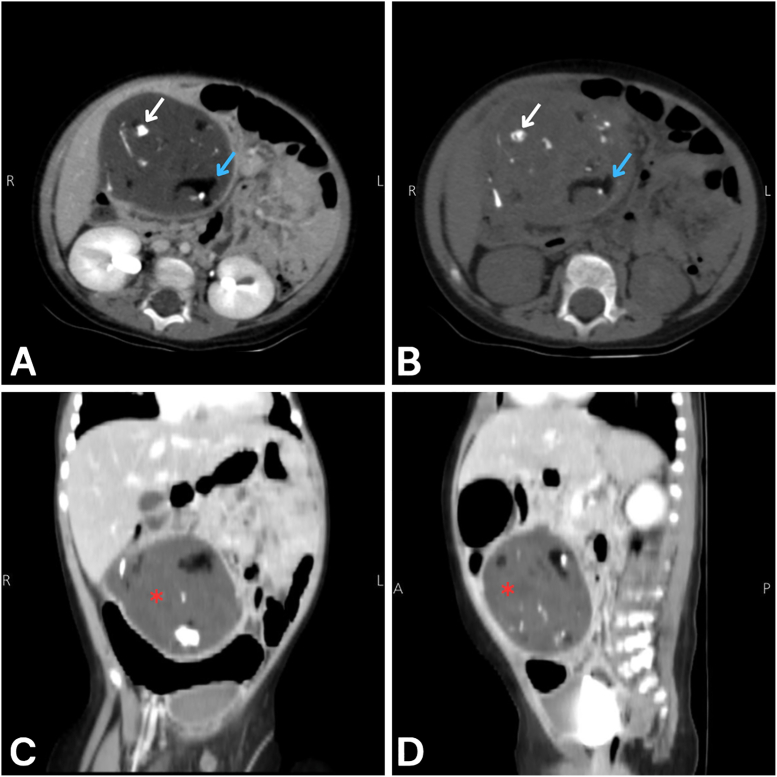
A: Axial abdominal CT without contrast. B: Axial abdominal CT with IV contrast. C: Coronal abdominal CT without IV contrast. D: Sagittal abdominal CT without IV contrast. The images show a large, well-defined, non-enhancing heterogeneous cystic mass (red asterisk) with areas of fat density (blue arrow) and coarse calcifications (white arrow), consistent with a testicular teratoma showing ischemic changes.
